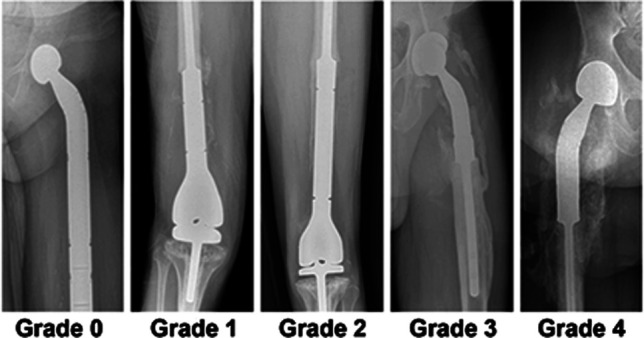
Radiographs demonstrating examples of grade 1 through grade 5 for the classification of heterotopic ossification around a femoral endoprostheses.
